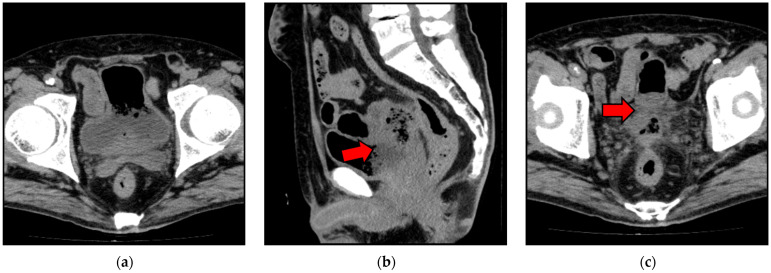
Residual debris-like shadows and intravesical gas accumulation in the urinary bladder (a). Although the fistula tract is not clearly delineated on CT, findings suggest a possible connection between the ileum and urinary bladder (b,c). Arrows indicate the suspected fistula region.
